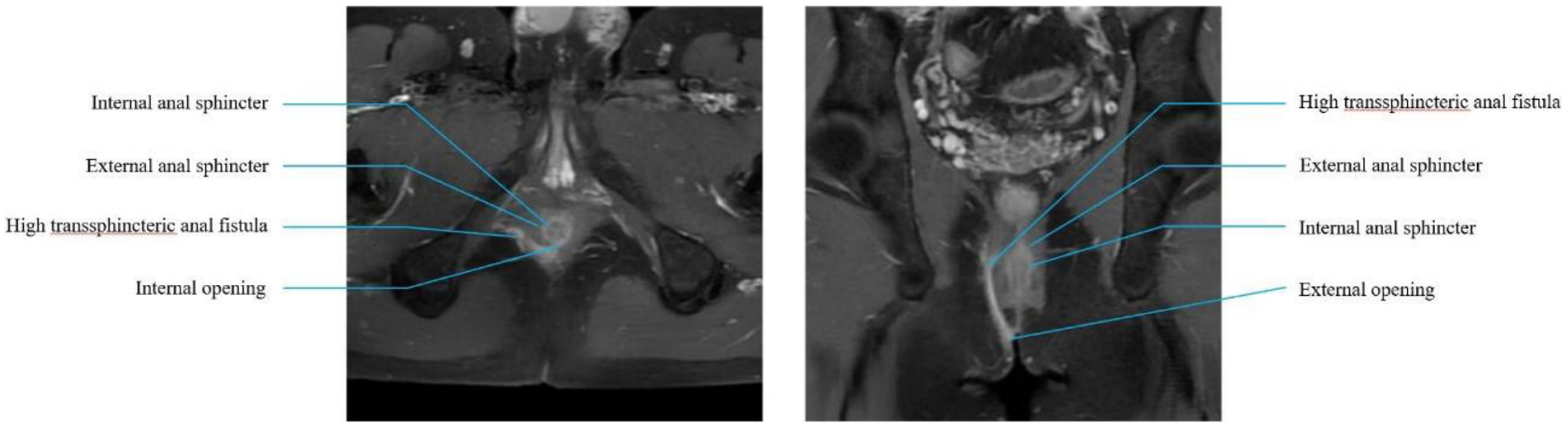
Preoperative MRI. Lithotomy and coronal section of preoperative MRI scan.
